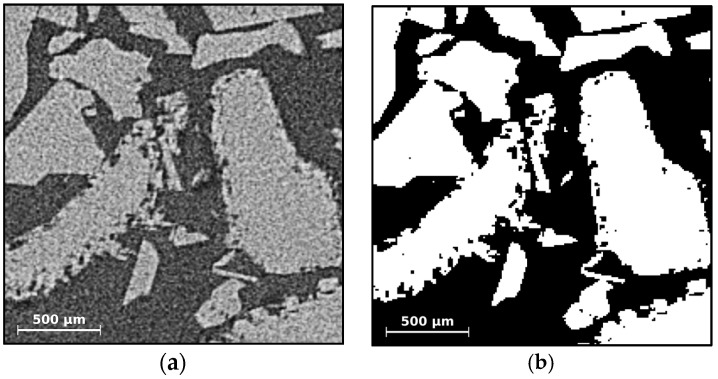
Segmentation of noisy CT images using the random walk segmentation filter described by Grady [52]: (a) Original CT slice taken from scan S5 of the THF clathrate experiment of Section 2.2.2. The bright phase is the THF clathrate, the dark phase is gaseous nitrogen; (b) The same slice after segmentation.
